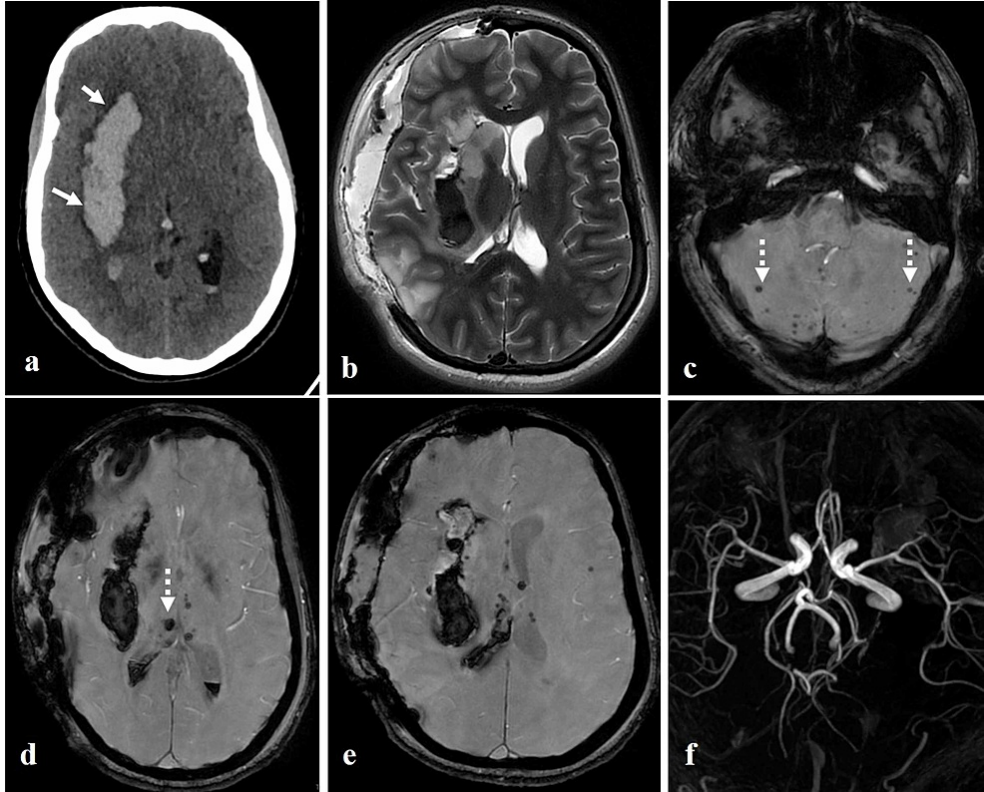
Non-contrast CT brain, post-operative magnetic resonance imaging (MRI) T2W image and MR angiography of brain. The axial non-contrast CT image (a) shows acute intraparenchymal hemorrhage at right gangliocapsular region with intraventricular extension (white arrows). The post-operative T2 image (b) shows right-sided fronto-parietal craniotomy with residual hemorrhage at the right basal ganglia. The axial SWI images (c, d, e) show multiple microhaemorrhage at bilateral cerebellar hemispheres, bilateral thalami, pons and left insular cortex (dashed arrows). The time of flight (TOF) MR angiogram (f) of cerebral vessels is normal.
